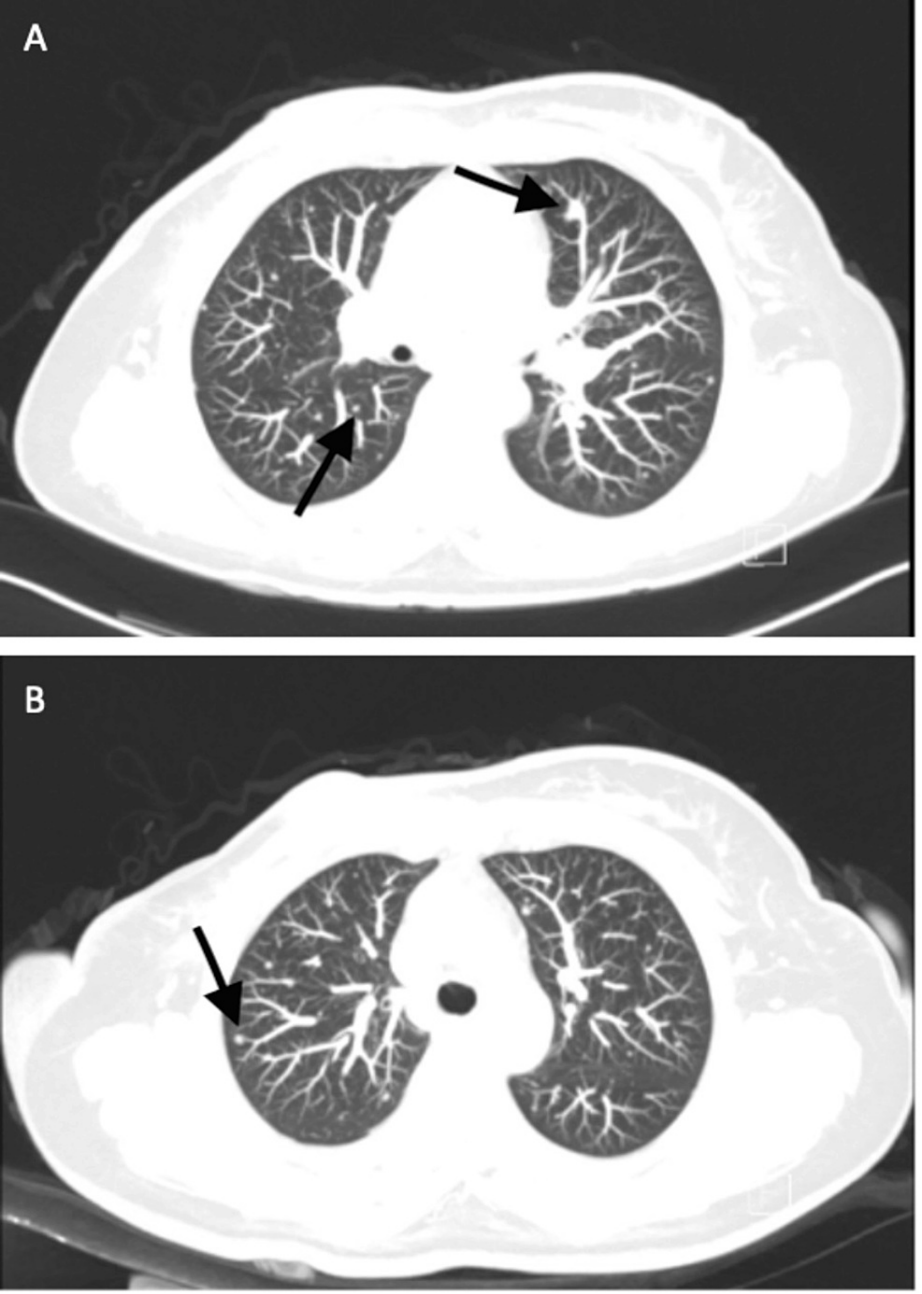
(A,B) Horizontal section of computed tomography showing widespread bilateral pulmonary nodules consistent with metastatic lung disease (arrows indicating some of the pulmonary nodules)
